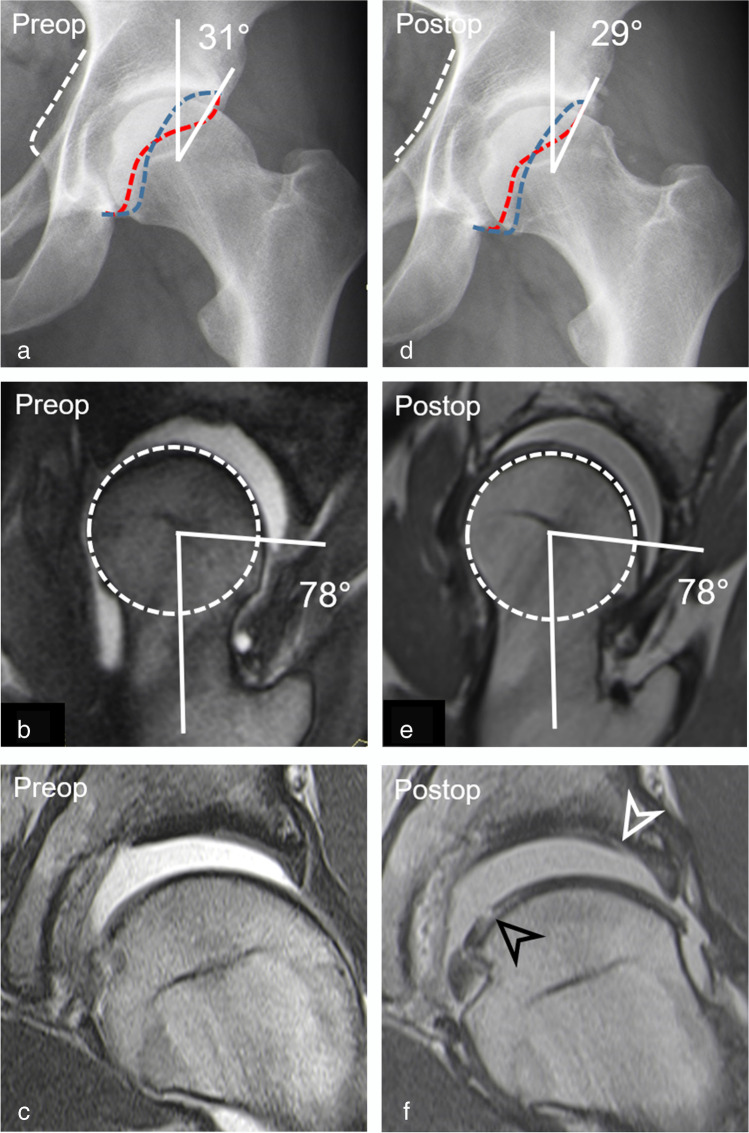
A-26 year-old man presenting with groin pain 3 years after arthroscopic acetabular rim trimming, labrum refixation, and cam resection. a Preoperative AP pelvis view shows acetabular retroversion with normal lateral coverage (LCE: 31°) and a cam deformity. b Preoperative radial reformatted image (3D T2-w true FISP, repetition time/echo time, 4.7 ms/2 ms) shows a cam deformity (alpha angle: 78°). c Preoperative coronal MR arthrogram (T1-w TSE sequence; 450 ms/ 12 ms) shows no chondral damage. d Postoperative radiograph shows less pronounced crossover sign. e Postoperative radial image (3D PD-w SPACE, 1100 ms/41 ms) shows incomplete cam resection with residual cam deformity postero-superiorly. f Postoperative coronal T1-w TSE image (450 ms/12 ms) shows progressive cartilage damage with new acetabular (white arrowhead) and femoral (black arrowhead) chondral damage. Note: Anterior acetabular wall (red dotted line), posterior acetabular wall (blue dotted line), ischial spine sign (white dotted line), femoral head (white dotted circle)
